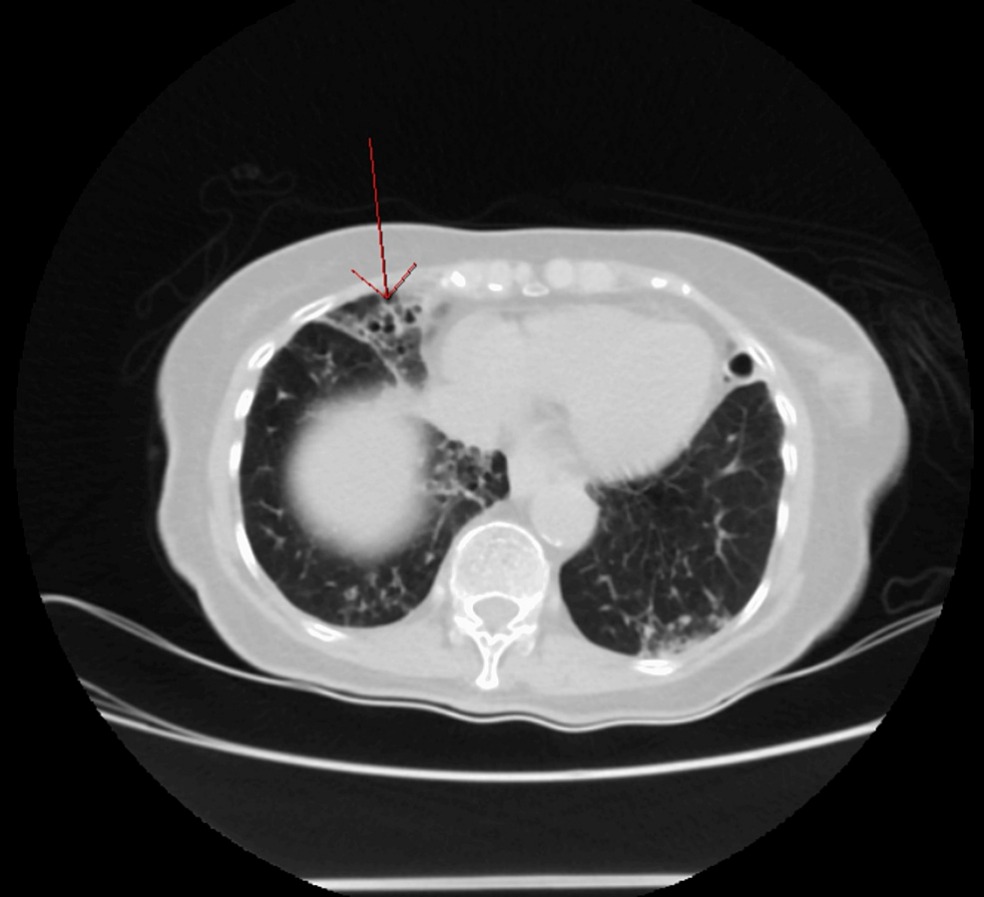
Nodular bronchiectasis involving the right middle lobe in the axial cut of CT abdomen/pelvis with intravenous contrast.
